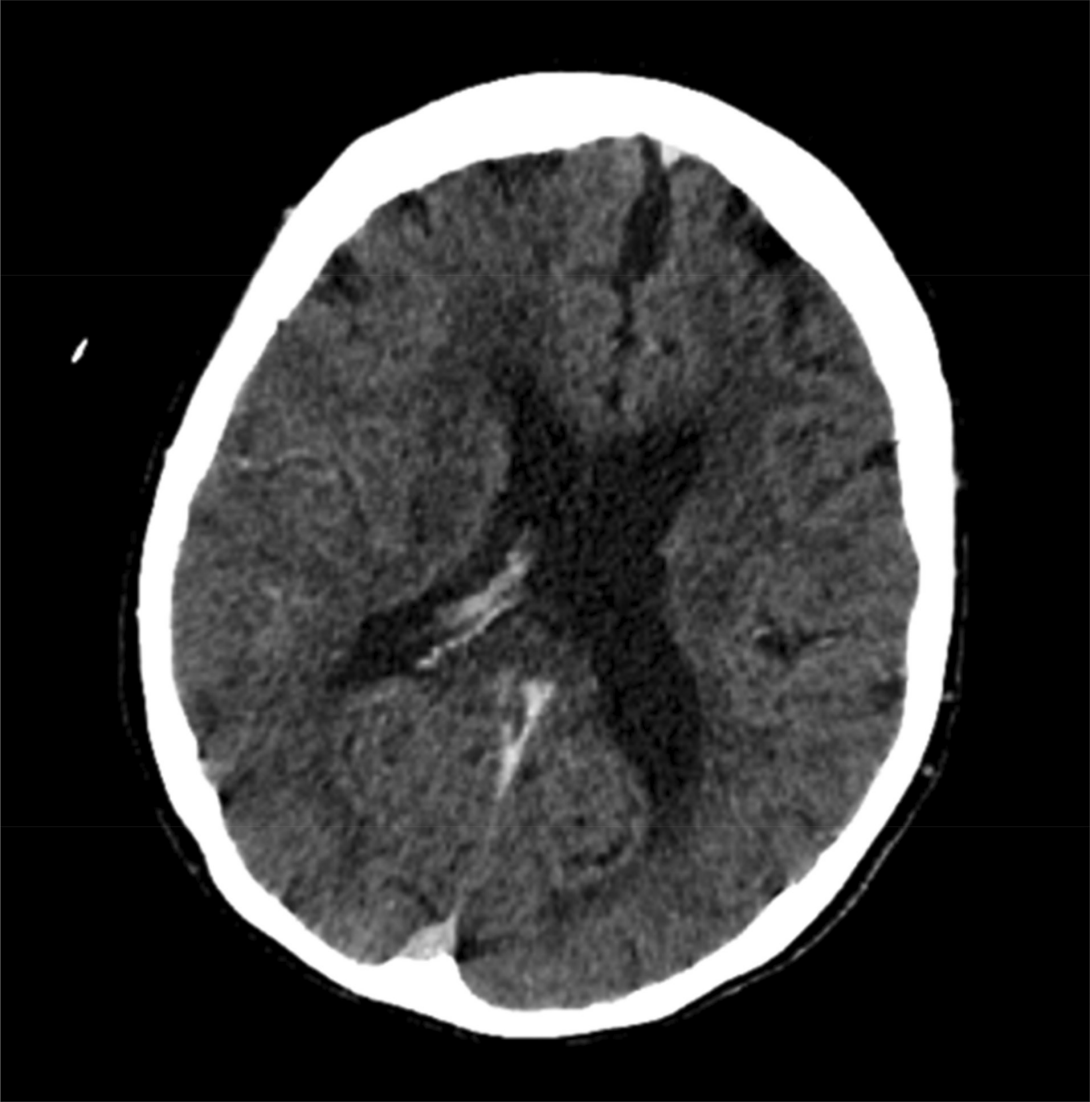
Repeat computed tomography (CT) with contrast demonstrating hypoattenuation in the right temporal lobe with loss of cortical medullary differentiation in the region of the right sylvian fissure, suspicious for an infective encephalitis.
